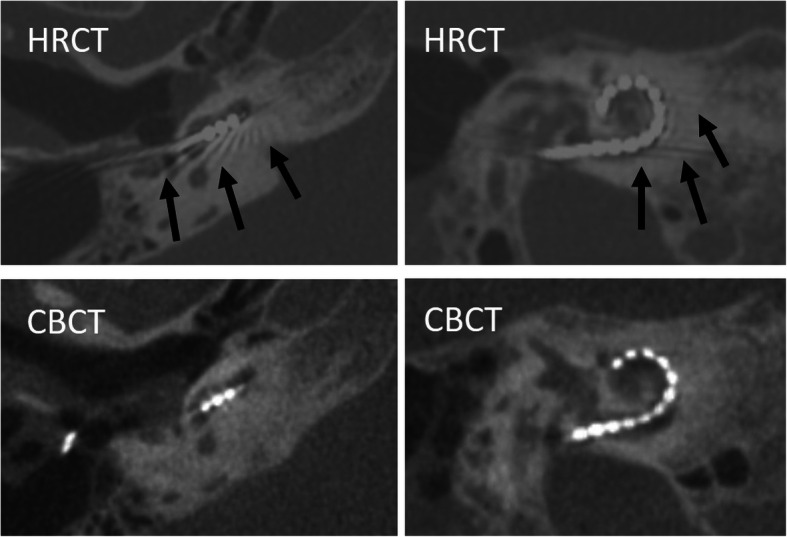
Comparison of postoperative HRCT and CBCT in a 53-year-old female patient. Axial (left images) and paracoronal (right images) images. HRCT images show more metal artifacts (arrows) and blooming artifacts than CBCT images. However, the scalar location and number of electrode contacts can be reliably documented in both modalities
